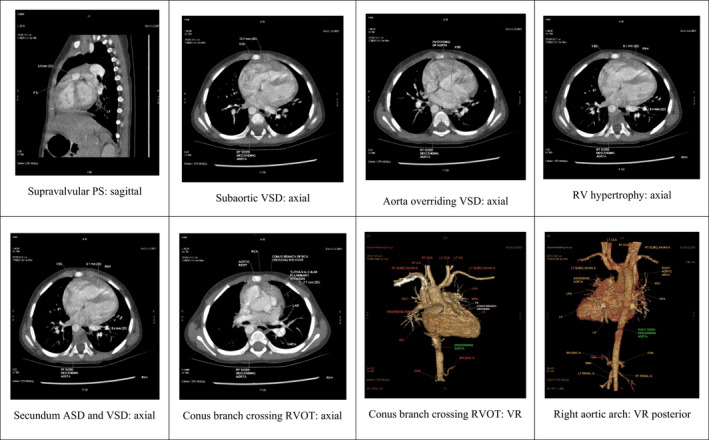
Cardiac CT scan of 1‐ year‐old male child, having Pentalogy of Fallot, right aortic arch, conus branch of right coronary artery crossing anterior to right ventricular outflow tract [RVOT] to left side with Pulmonary Vein Index [PVI] of 368.12 mm^2^/m^2^, McGoon Ratio of 1.97, and Nakata Index of 539.22 mm^2^/m^2^
